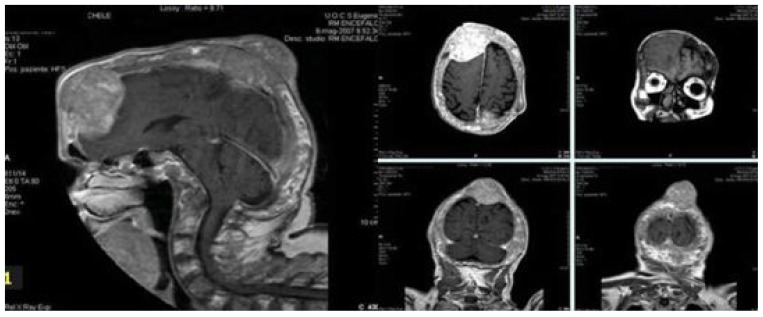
Cranial MRI. Evident eso-endophytic mass lesions through the skull with contrast enhancement and brain parenchima compression.
